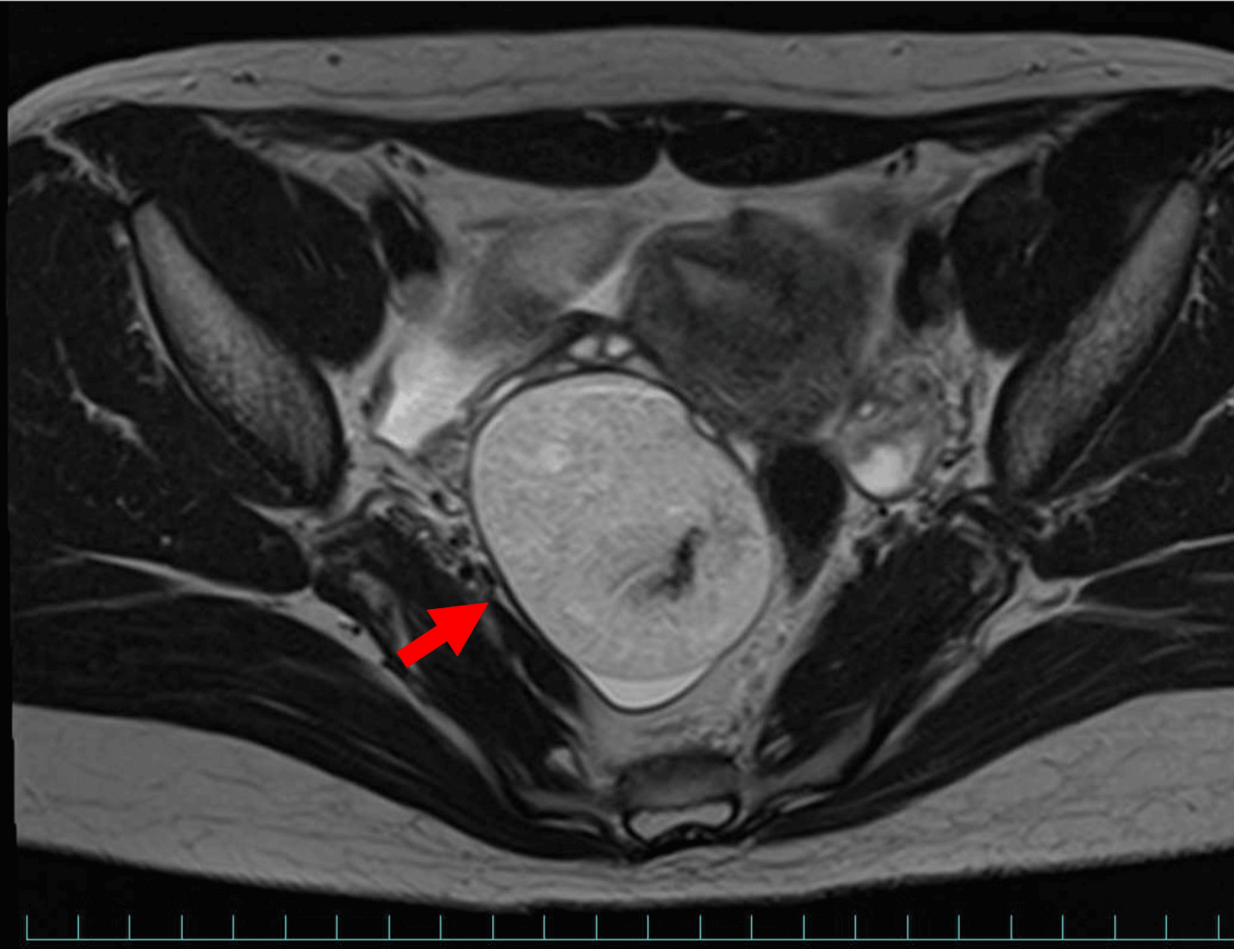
MRI of mature cystic teratoma on the right ovary. The red arrow indicates a 6 cm mature cystic teratoma on the right ovary.
